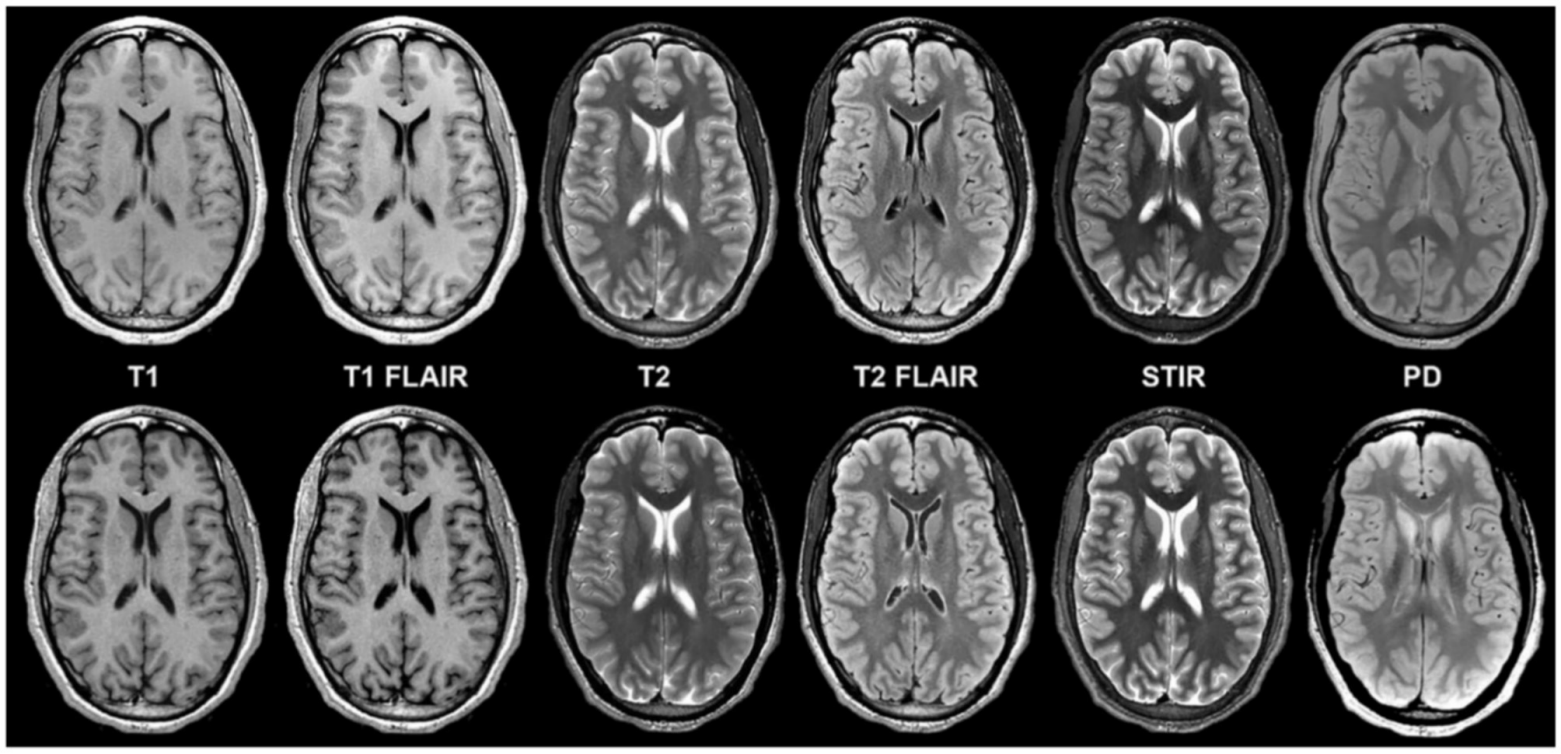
Example of a “MAGiC” sequence of synthetic MRI, in which a standardized set of sequences is constructed from a single scan, the total time of acquisition being about 5 minutes. The sequences in the upper row are conventionally acquired, while those in the lower row are synthetic (24). Reprinted from Tanenbaum et al. (36).
